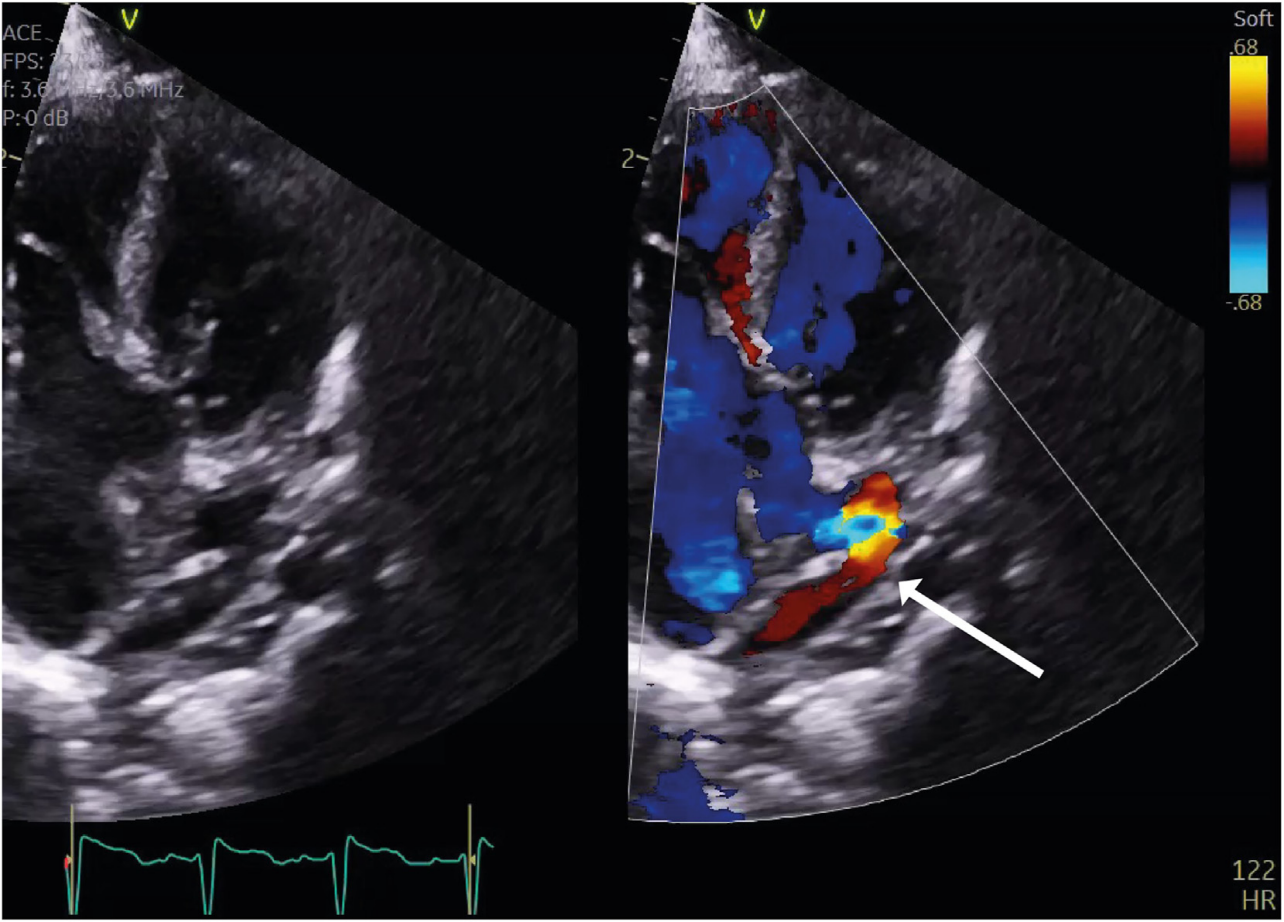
Two-dimensional transthoracic echocardiogram, obtained 2 months after TAPVC repair and PA band, apical four-chamber end-diastolic view without (*left*) and with (*right*) simultaneous color flow Doppler, demonstrates narrowing of the confluence anastomosis.
